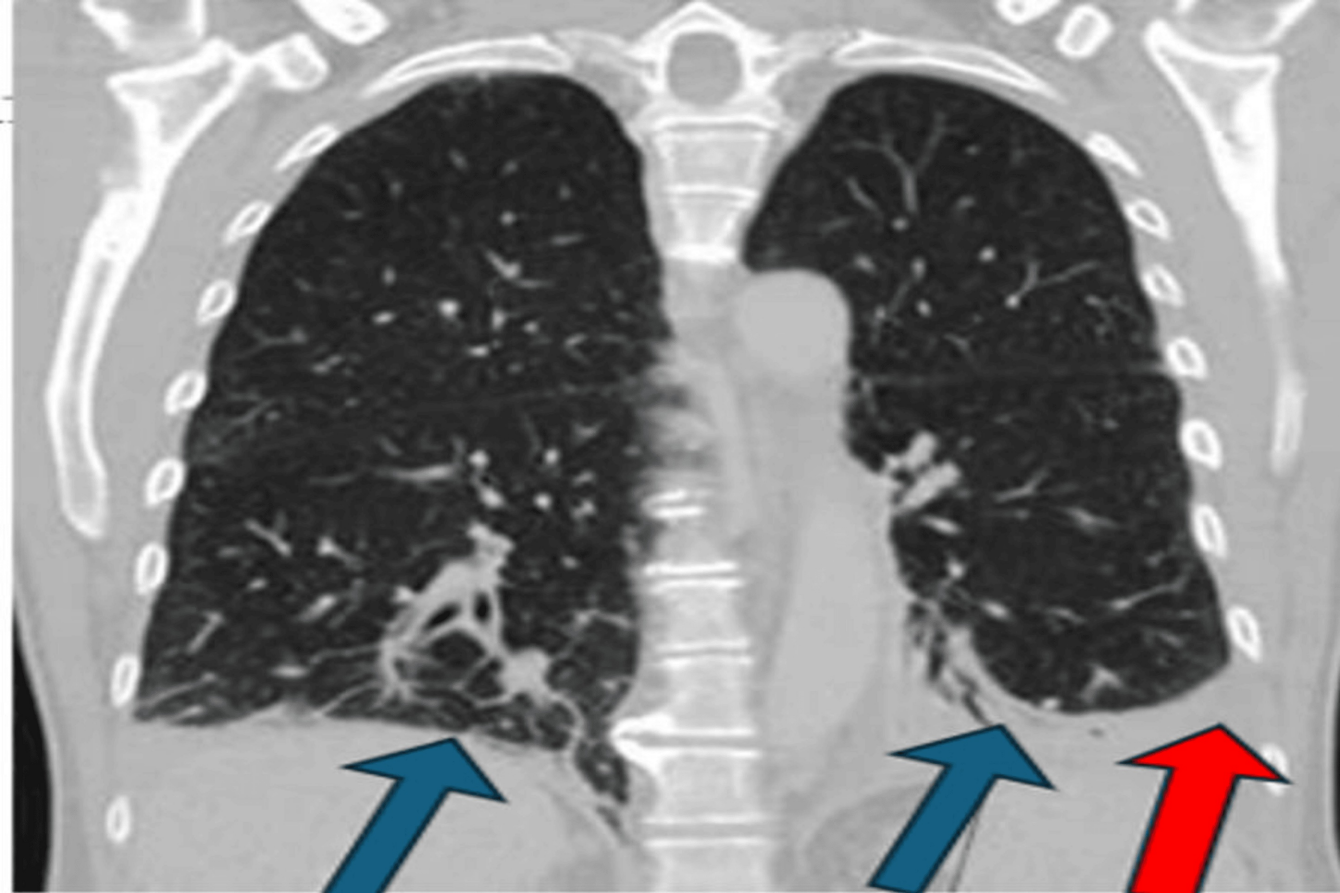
Chest CT scan reveals new-onset bibasal consolidation (blue arrows) and a mild left-sided pleural effusion (red arrow).
